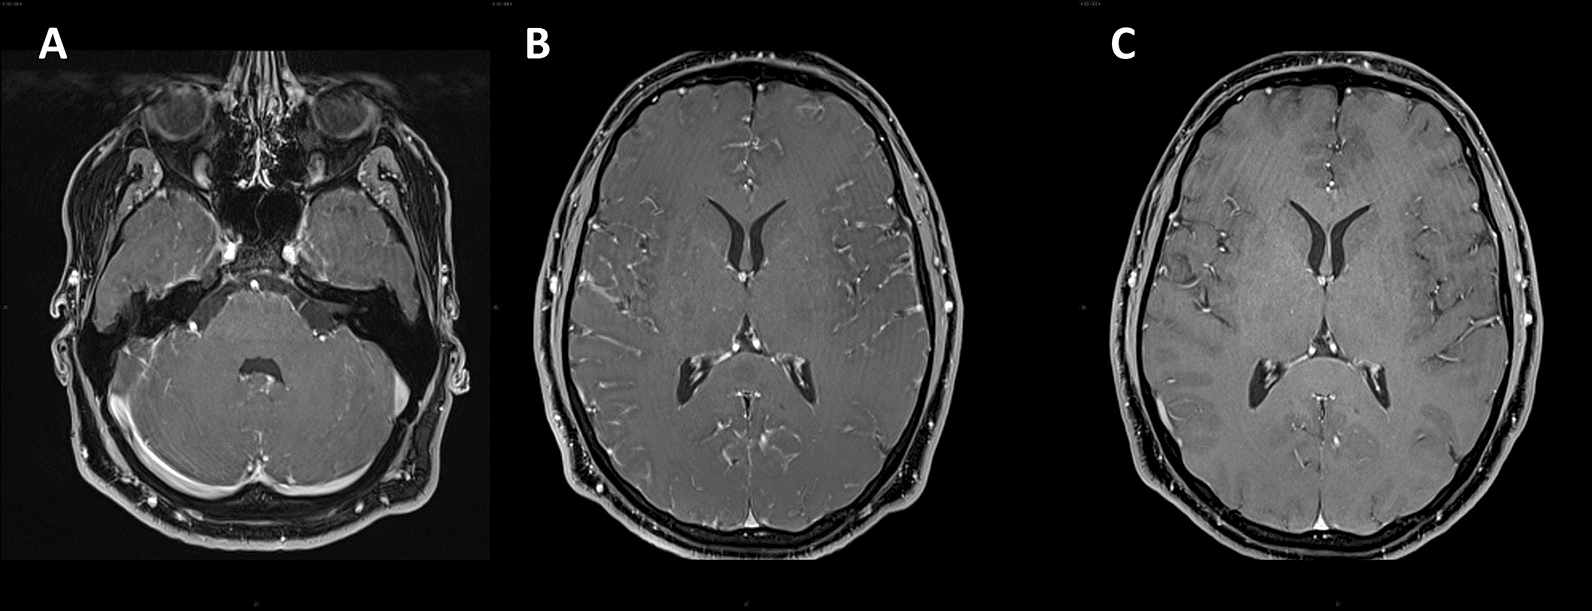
MRI shows diffuse leptomeningeal enhancement (subtentorial, A; supratentorial, B); Figure C
